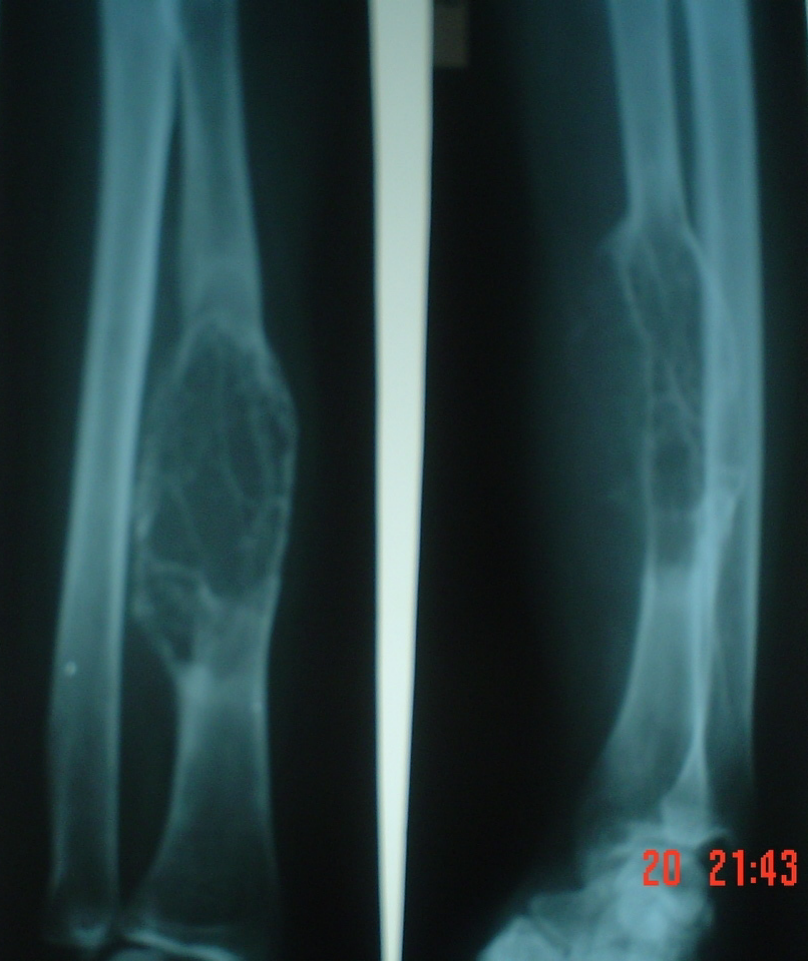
Radiograph of diaphyseal giant cell tumour.
